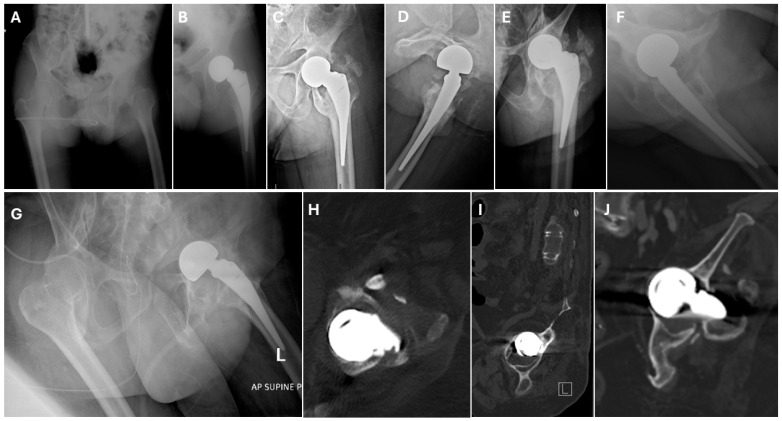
(A) The plain hip X-rays of a 47-year-old man with a displaced left femoral neck fracture. (B) Postoperative plain hip radiographs taken immediately following cementless bipolar hemiarthroplasty. (C,D) Twenty-two-month postoperative plain radiographs and CT scans of the pelvis, showing HO and radiographic acetabular erosion. (E,F) Postoperative plain hip radiographs at 61.6 months, showing HO and acetabular erosion progression. (G–J) X-rays and CT scan images taken approximately 177 months postoperatively (axial [H] and sagittal [I], and coronal [J]) showing an almost completely fused hip with class 4 HO and acetabular protrusion.
